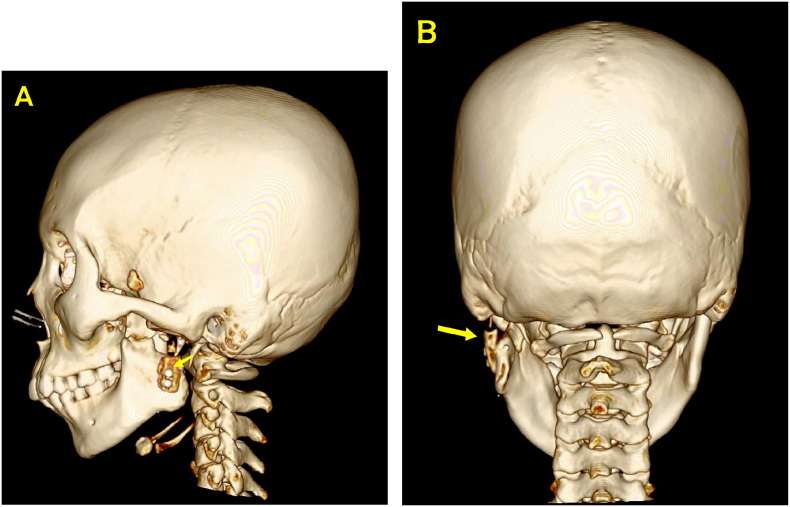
A and B 3D CT image of the head 3 months postoperatively. It showed the costochondral graft was well fixed on the ramus (yellow arrow). (For interpretation of the references to colour in this figure legend, the reader is referred to the web version of this article.)
